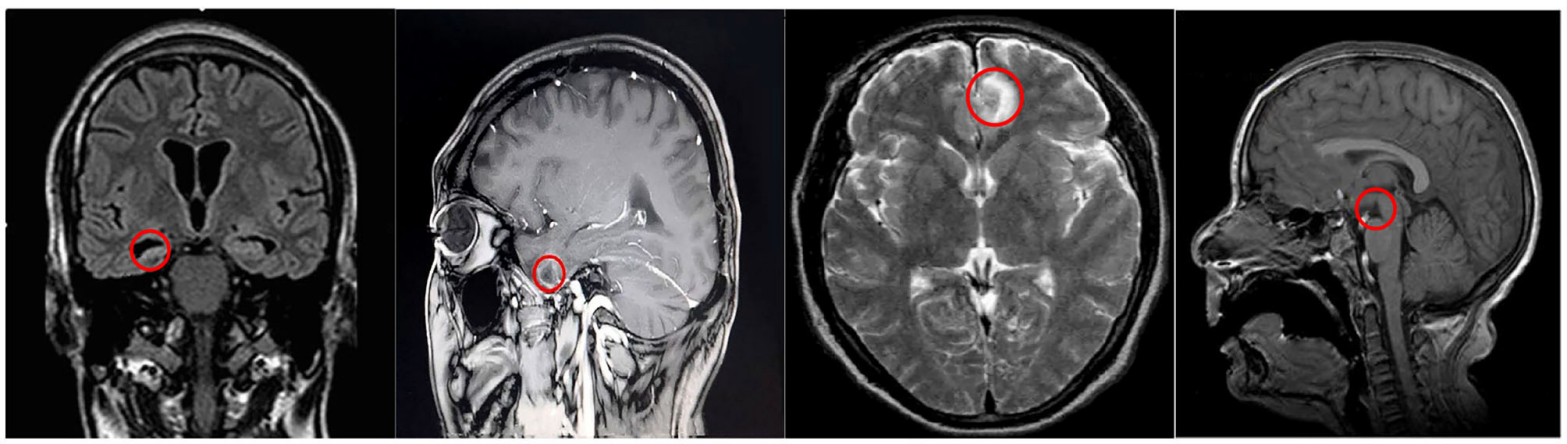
MRI images of refractory epilepsy in children brain.
